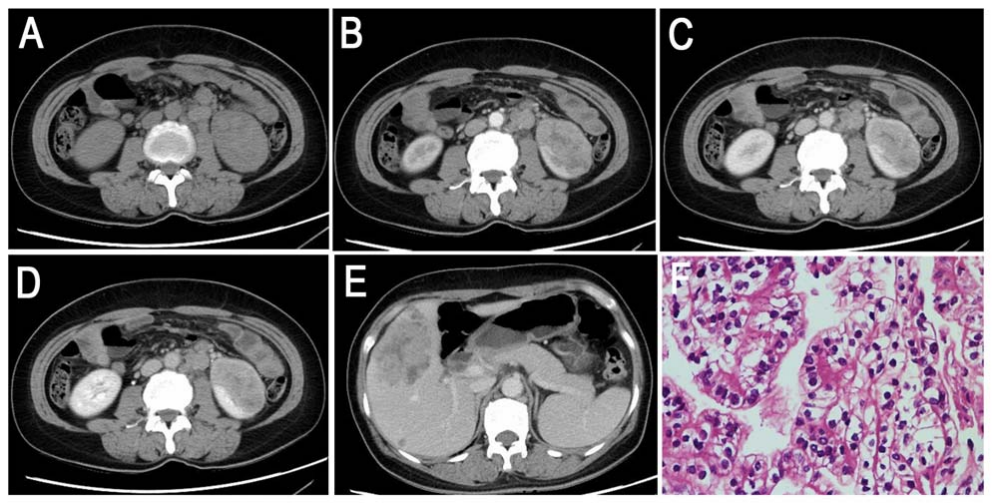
Translocation RCC in a 40-year-old woman (patient 10 in Tables 1, 2, 3) complaining of left flank pain for approximately half a year that became more serious 1 month prior to imaging. A, Axial unenhanced CT image showing an ill-defined, slightly higher attenuation soft tissue mass in the left kidney. Retroperitoneal adenopathy is present. B–D, Axial CMP, EP and NP contrast-enhanced CT images demonstrating moderate and prolonged enhancement of the mass without an integrated capsule. E, Liver metastasis with hemorrhage on EP is observed. F, The tumor cells consist of poorly differentiated clear cells (HE 10 & 40).
